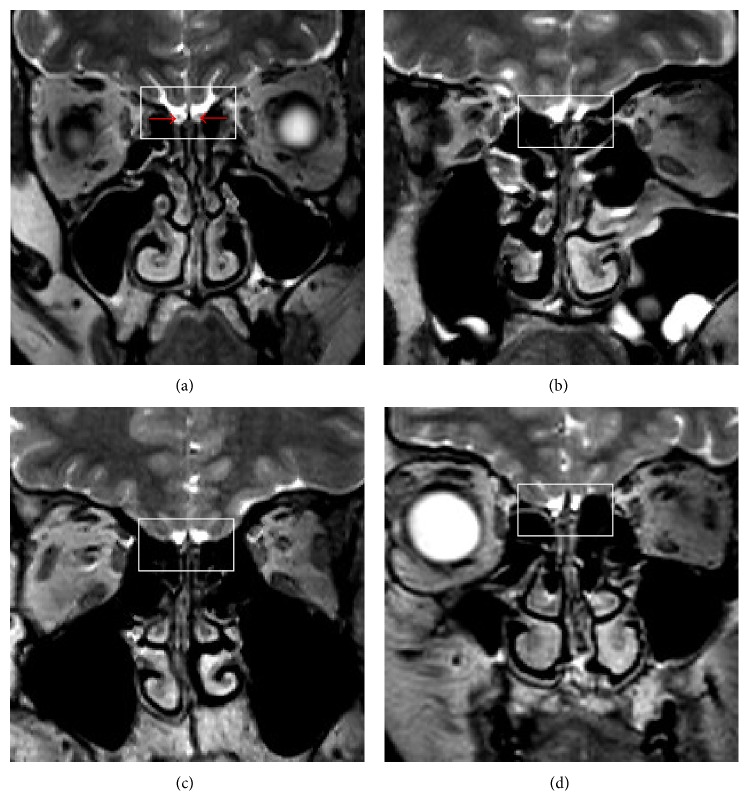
Magnetic resonance imaging (MRI) results of the three Kallmann syndrome males with cleft lip/palate. (a) T2-W MR images show normal structures in a normal control male. The red arrows indicate olfactory bulbs. ((b), (c), (d)) The MRI showed the lack of the bilateral olfactory bulb, the olfactory tract, and sulcus (squares) in cases 1, 2, and 3, respectively.
